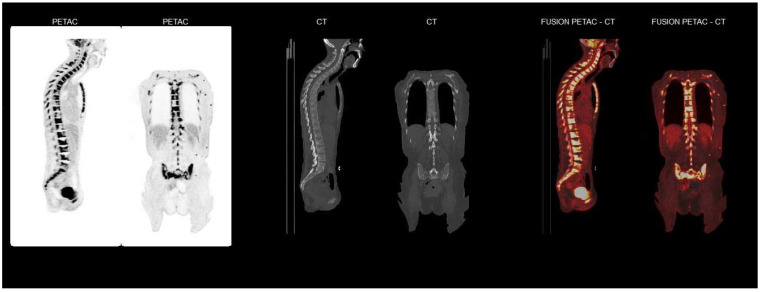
[^18^F]FDG-PET/CT of the vertebral column/bone marrow. The pictures demonstrate extensive FDG-uptake in the whole axial skeleton.
